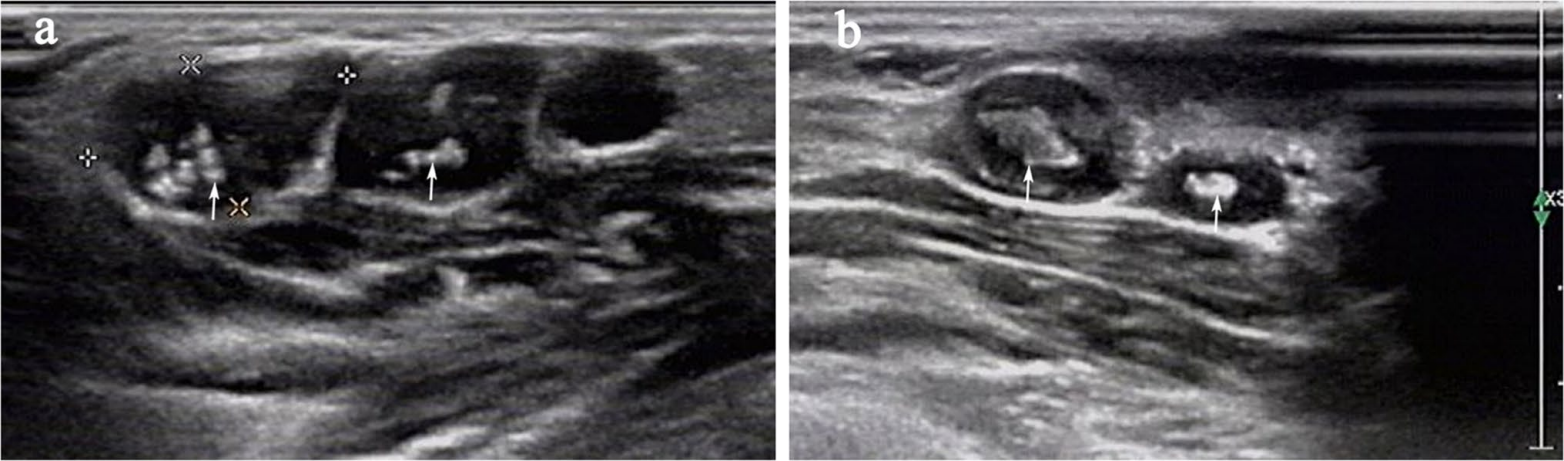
Cervical ultrasonography of the lymph nodes (LNs) shows strong echo. a Multiple strong echoes were seen within two of three lymph nodes lined up (arrows); b strong echoes were seen within two enlarged LNs (arrows)
